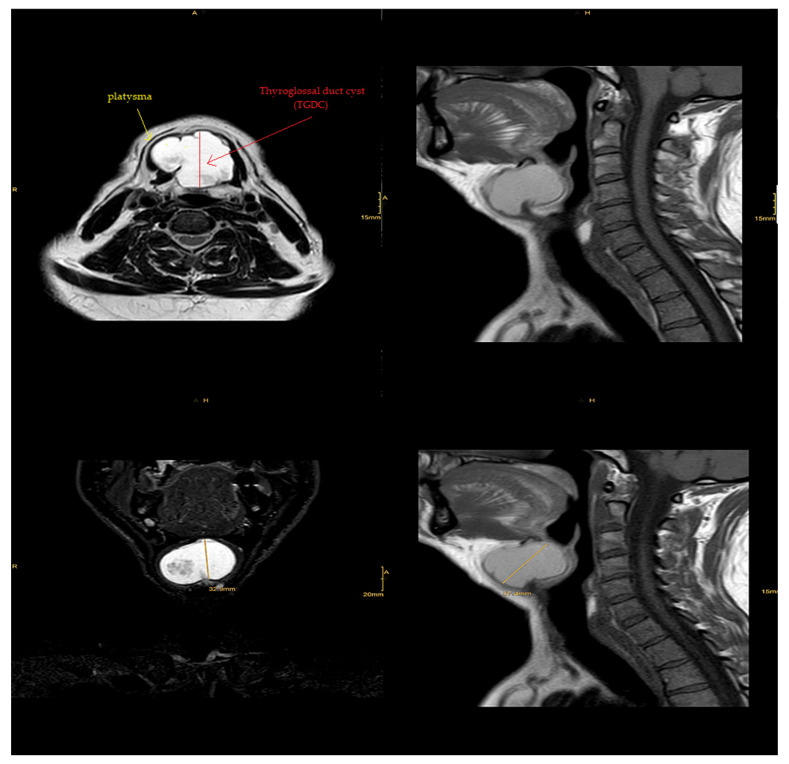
MRI image of the neck’s soft tissues (native and IV KM) showing suprahyoid thyroglossal cyst located posterior to the platysma.
